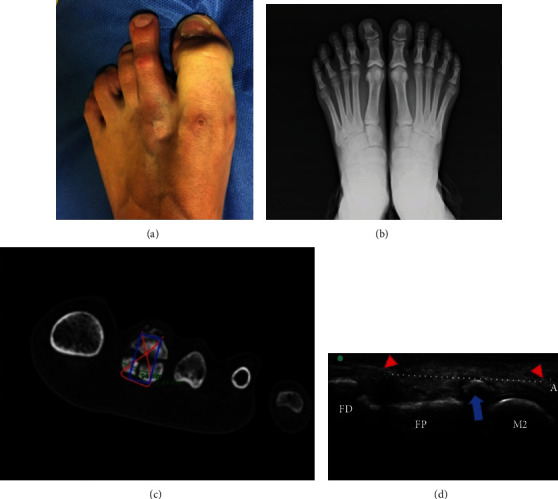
(a) Weakness of the second toe to due to flexor rupture. (b) Anteroposterior weight-bearing X-rays. (c) Preoperative planning on CT scan. (d) Longitudinal plantar sonographic view (M2: head of second metatarsal bone; FP: proximal phalanx; FD: distal phalanx; blue arrow: stress avulsion fracture of the FP; red triangles: flexor rupture).
